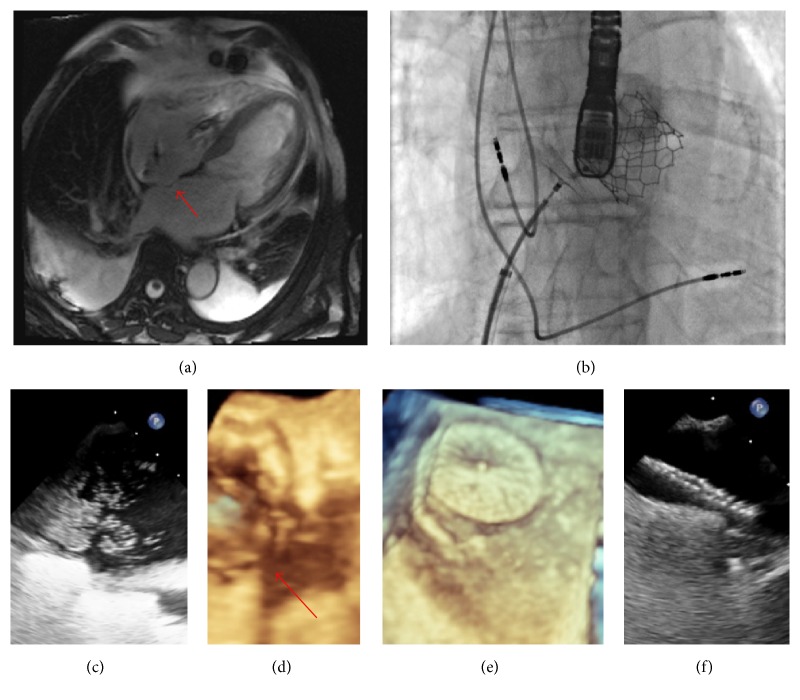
Platypnea-Orthodeoxia Syndrome after transcatheter aortic valve implantation. (a) Cardiac Magnetic Resonance 4-Chamber Image from 4 years earlier, showing thinned and bulging interatrial septum (red arrow) with no evidence of aneurysm or transseptal contrast flow, right ventricular thickness = 7 mm, and mild tricuspid incompetence with no evidence of carcinoid infiltration. (b) Fluoroscopy imaging showing 35 mm septal occlusion device in situ before deployment, with Edwards S3*™* 29 mm transcatheter aortic valve device and transoesophageal probe visible. (c) Intraoperative transoesophageal contrast study showing a large shunt from right-to-left atrium, possibly with the presence of more than one fenestrated defect. (d) Three-dimensional transoesophageal image of defect (red arrow) and transcatheter aortic valve in situ. (e) Three-dimensional transoesophageal showing PFO device in situ. (f) Postprocedure negative bubble study with device in situ.
